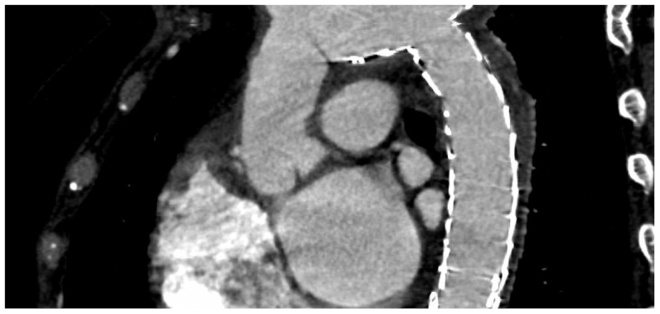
Pre-repair and fistula development computed tomography (CT) image. Centerline image of patient's chest status post ascending aorta/aortic arch graft repair, aortic debranching, and thoracic endovascular aortic repair (TEVAR) of the descending thoracic aorta for aortic dissection repair.
